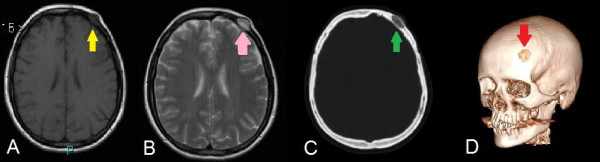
A magnetic resonance imaging scan shows a hypointense lesion (yellow arrow) on the T1-weighted image (A) and a hyperintense lesion (pink arrow) on the T2-weighted image (B); a computed tomography scan reveals an osteolytic lesion (green arrow) with erosion of the tabula externa (C); a three-dimensional reconstruction of the skull with evidence of the lesion in the pre-operative time (red arrow) (D).
